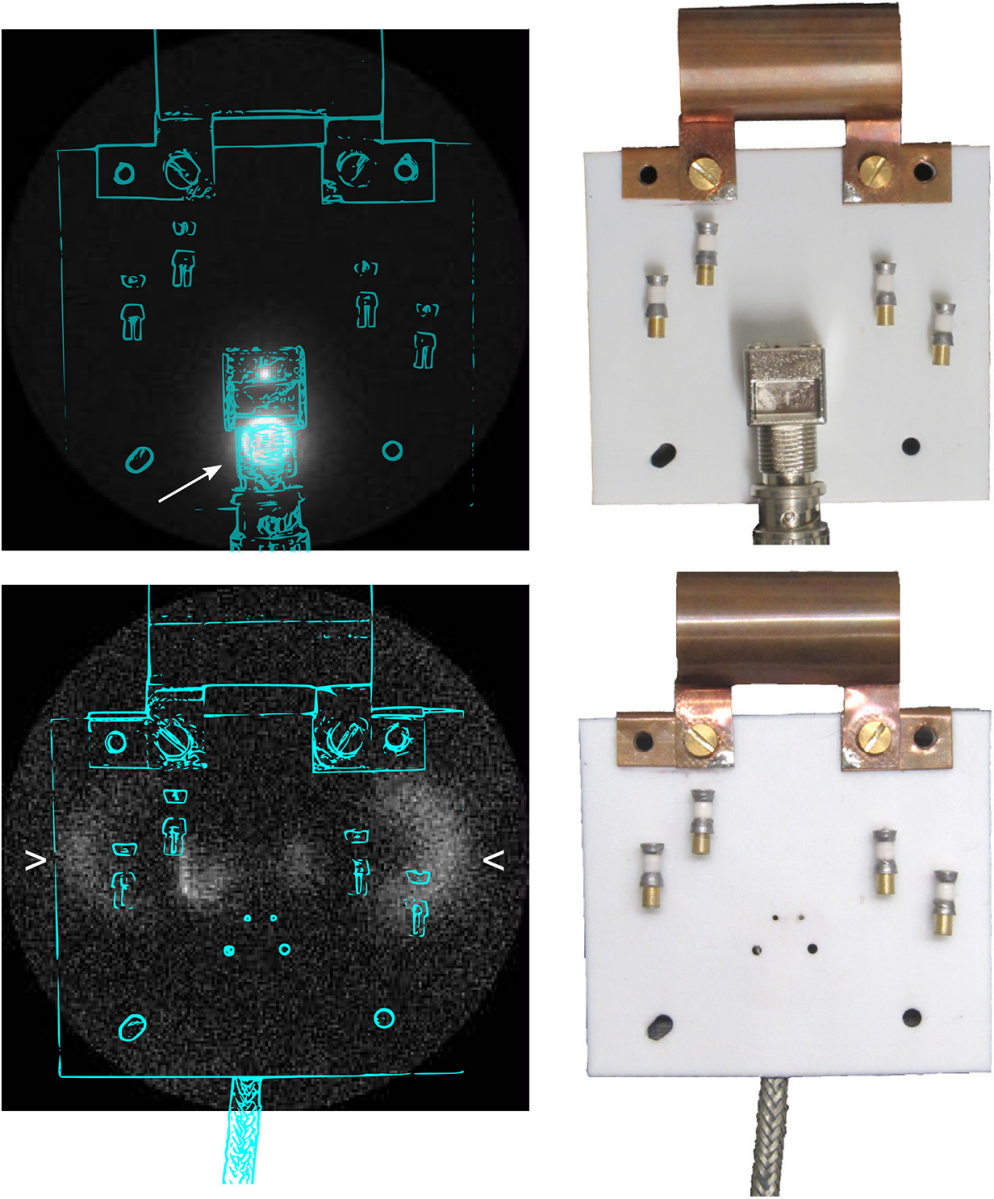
3D maximum-intensity-projection ZTE MRI and approximate coil positions (left) acquired at 9.4 T and photograph of coil assembled from CB_2_ and LG_2_ (right), either with a conventional BNC connector and coaxial cable (top), or a custom-made PTFE cable that was soldered to the circuit board (bottom). Note that the signal originating from the BNC connector was removed using the custom build cable. In turn, signal originating from the variable capacitors appeared close to the noise level (FOV = 20 cm).
